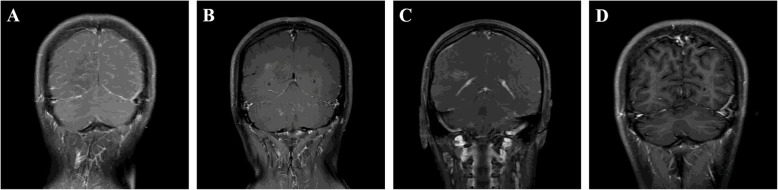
Serial coronal T1 postcontrast MRI at presentation and follow-up. Coronal contrast-enhanced MRI (A) revealed diffuse leptomeningeal enhancement at presentation. Repeated enhanced-MRI after 3 months (B) showed enhanced lesions in the cerebellum, corona radiata, frontal and parietal white matter. MRI at 4 months from presentation (C) showed linearly vessel enhancement in the region of the ependyma. No enhancement was found on enhanced-MRI at 6 months from presentation (D)
